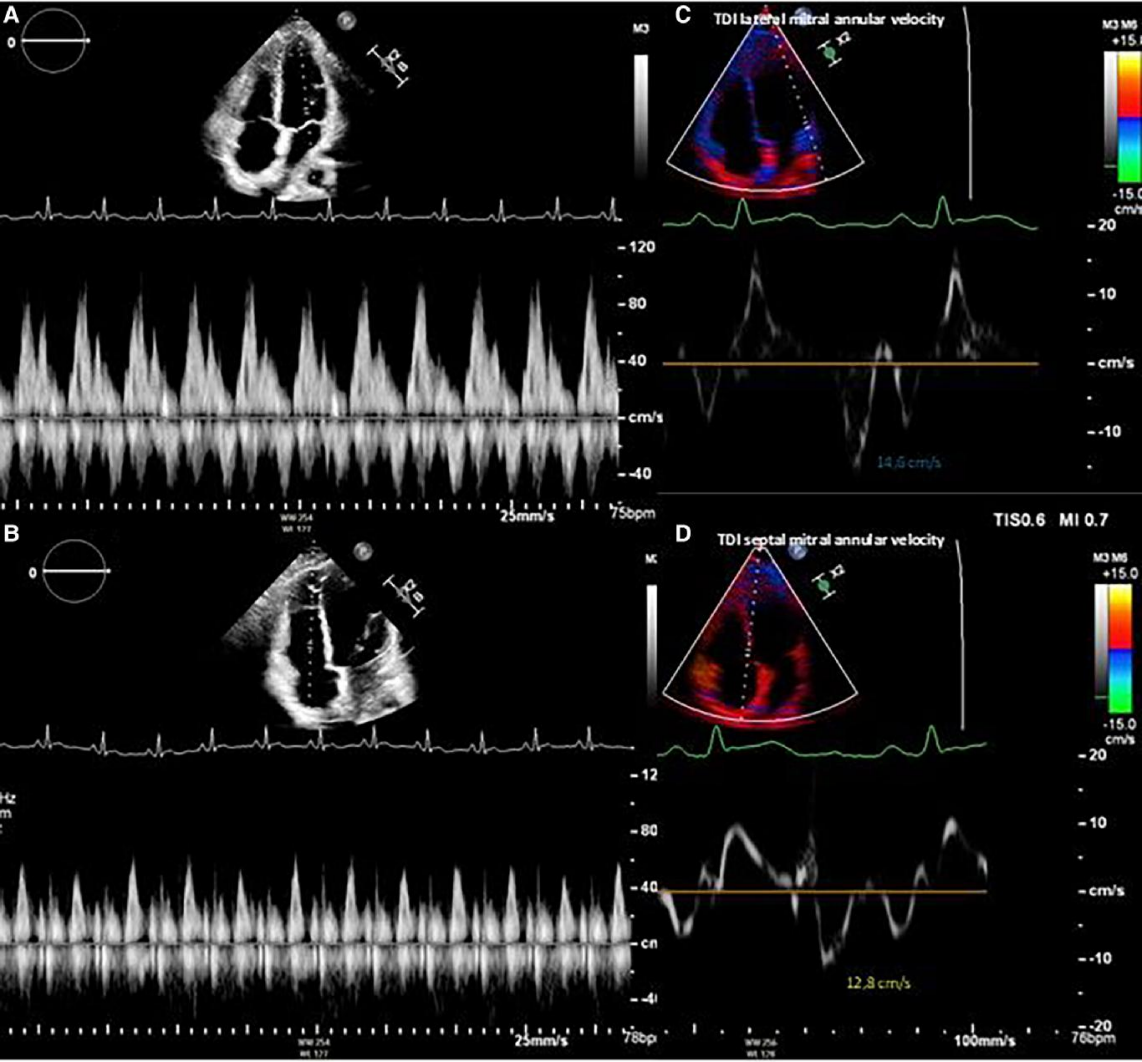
Echocardiography (*A–D*) showing the absence of constrictive pattern after IL-1R antagonist treatment.
